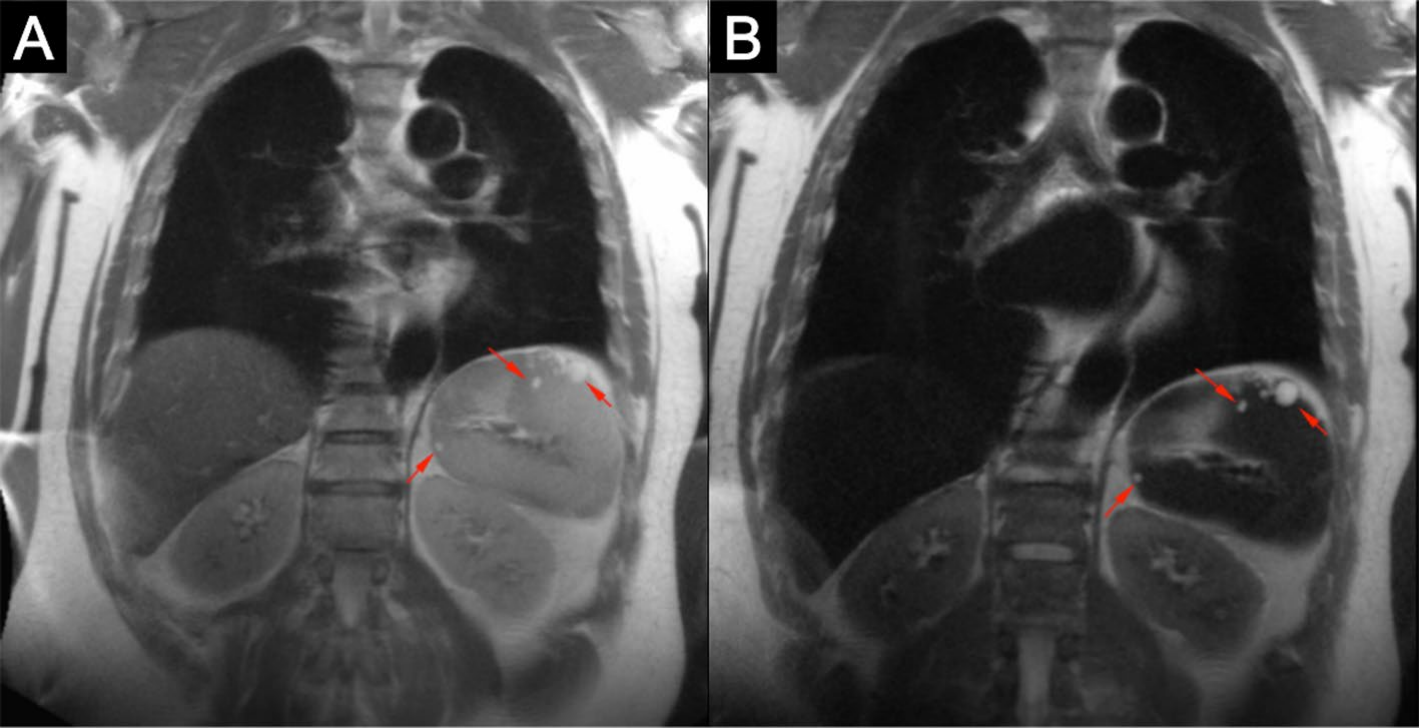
3.0 T ferumoxytol-enhanced MRI in a 60-year-old male with multiple splenic cysts. Pre- (a) and post-contrast (b) T2 acquisitions show increased conspicuity multiple benign cysts (red arrows) so that even subcentimeter cysts can be distinguished from the normal splenic tissue that loses signal with the addition of ferumoxytol
